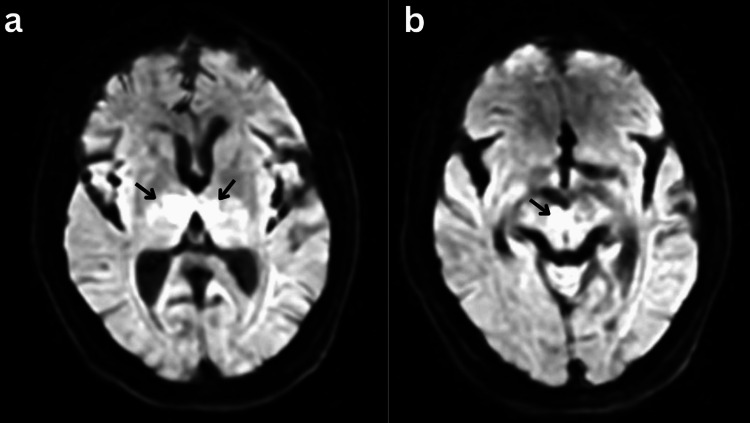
Magnetic resonance diffusion-weighted imaging (DWI) a) Bilateral thalamic and b) Right-sided rostral midbrain restriction.
